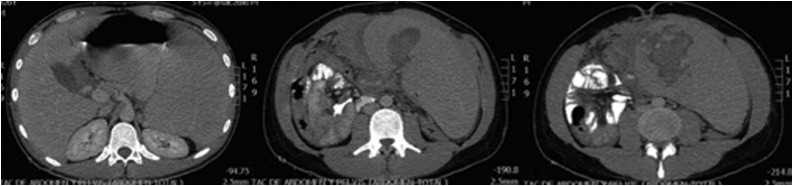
Abdominal CT scan, axial slices at T12 (left), L2 (middle) and L3-L4 intervertebral disc (right). Patient data was deleted.
